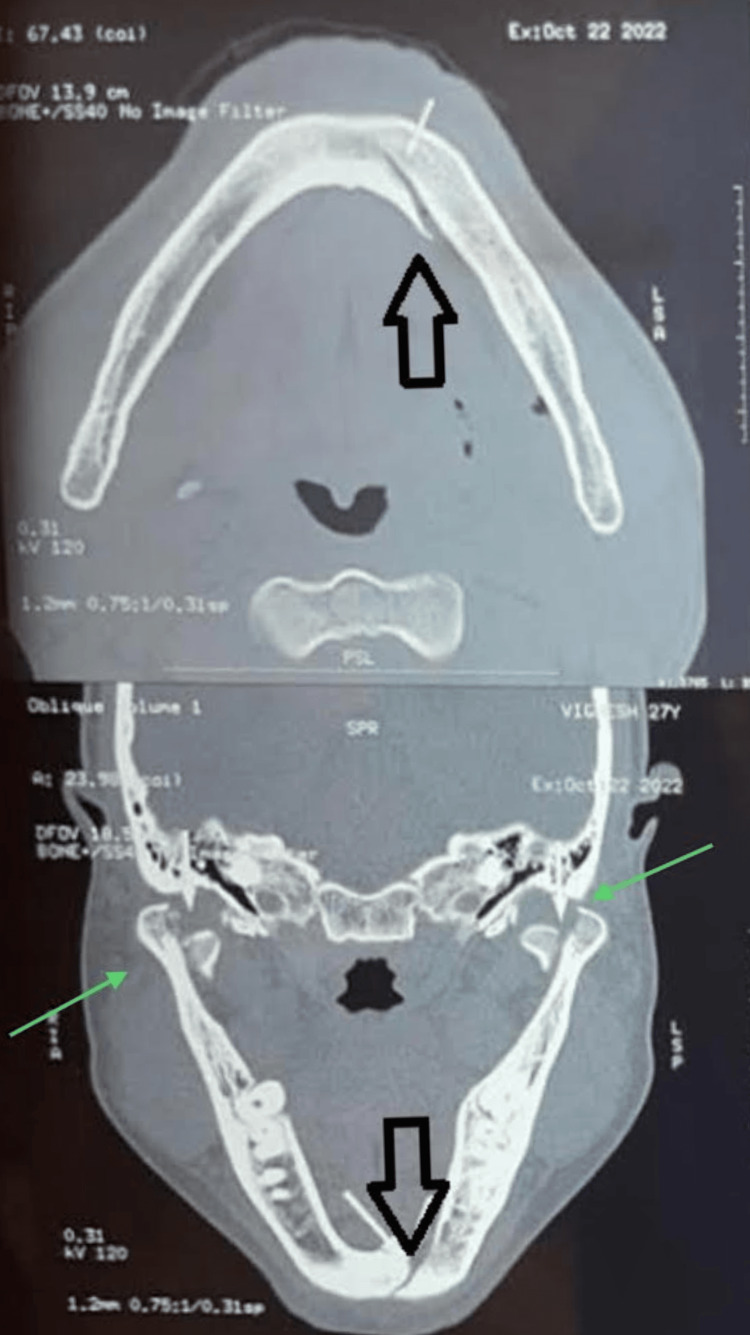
Pre-operative radiograph This image shows a pre-operative radiograph showing symphysis and a bilateral dicapitular condyle fracture.
